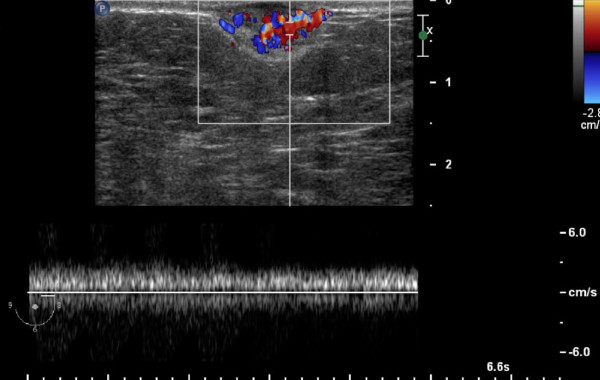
Ultrasonography. Highly vascular lesion in the Doppler mode.
